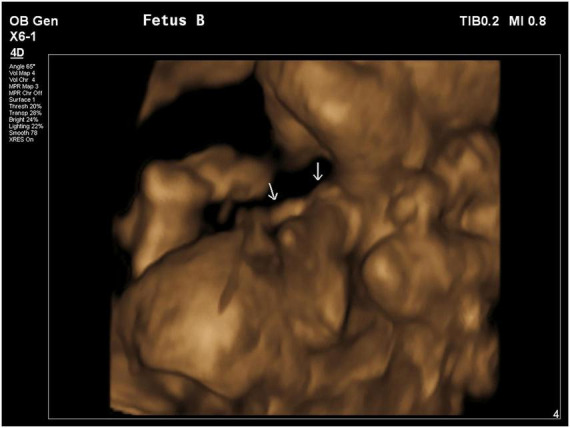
Three-dimensional ultrasound image of the fetus. The white arrow indicates the fetal right ankle, with faint umbilical cord signs visible around it.
